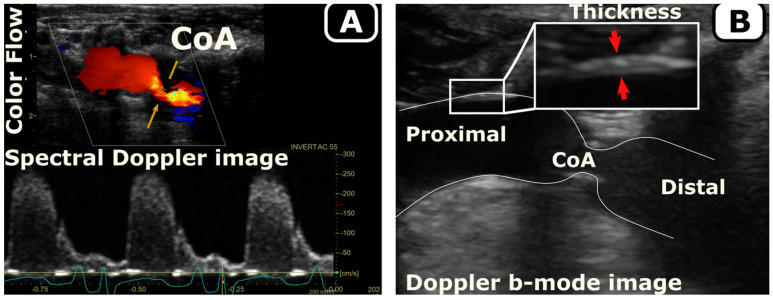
Quantification of hemodynamics (A) and morphology (B) in a rabbit model of CoA. Doppler ultrasound follow-up was performed weekly to quantify the temporal evolution of hemodynamic changes (A, spectral Doppler) and morphology (B, Doppler b-mode) in response to the CoA.
